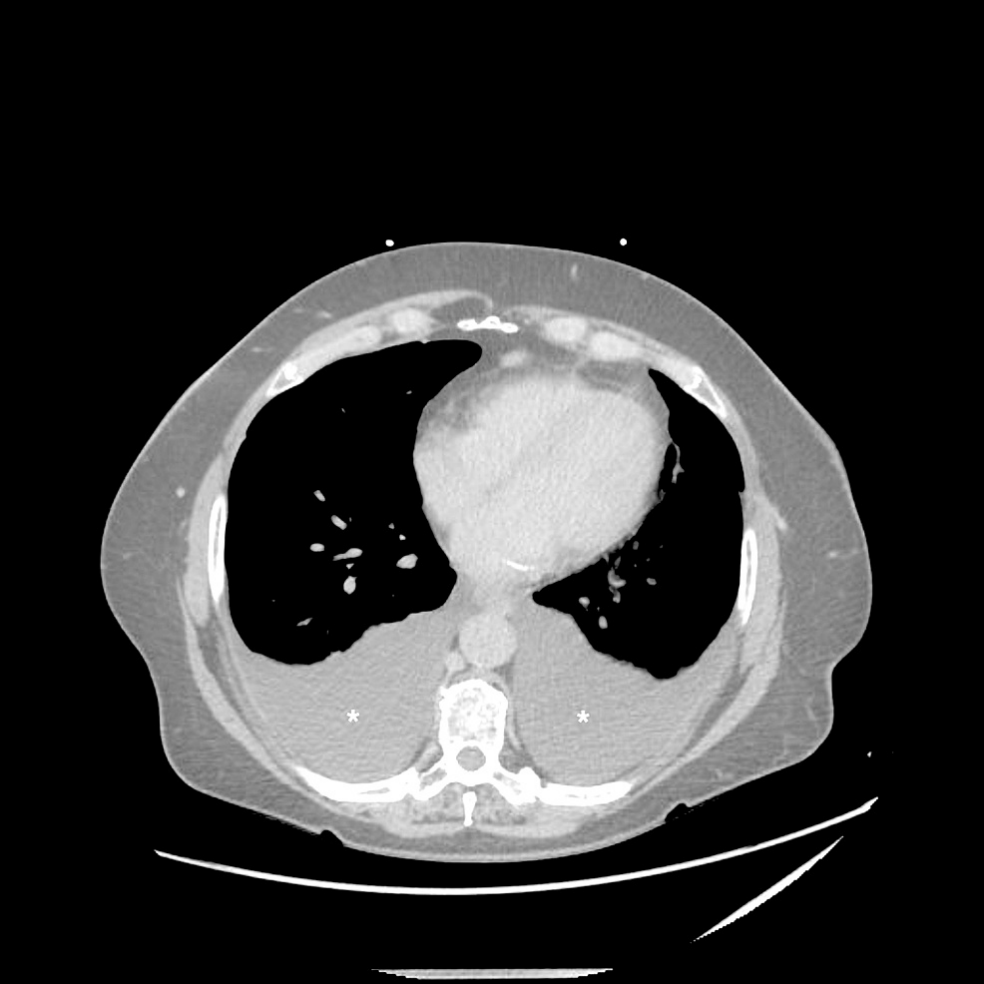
Computed tomography of chest (axial section) CT chest demonstrates large bilateral pleural effusions (white asterisks)
